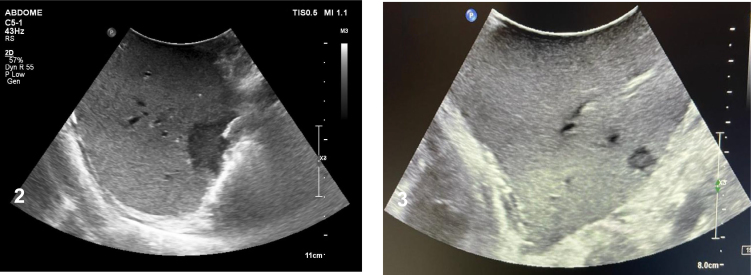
Intraoperative ultrasound lesions on segments VII and segment II.
